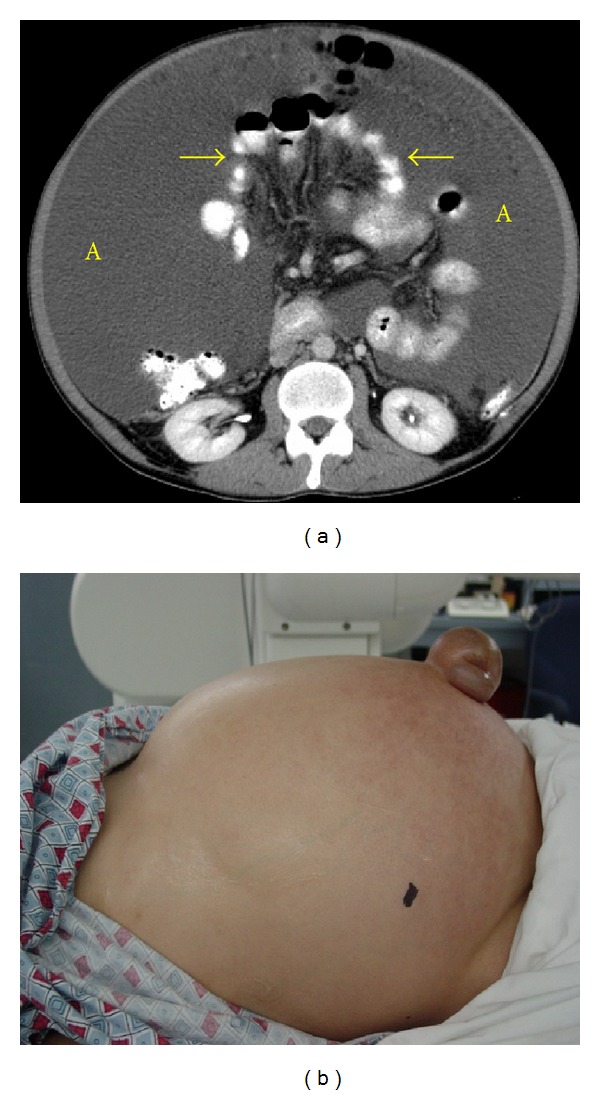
Tension ascites in a cirrhotic patient. (a) An axial contrast-enhanced CT demonstrates a large intraperitoneal low density fluid collection (A). Central displacement of the bowel and mesenteric structures (arrows) commonly seen in patients with benign intraperitoneal fluid (transudate). (b) Photograph of the patient's abdomen before decompressive paracentesis.
